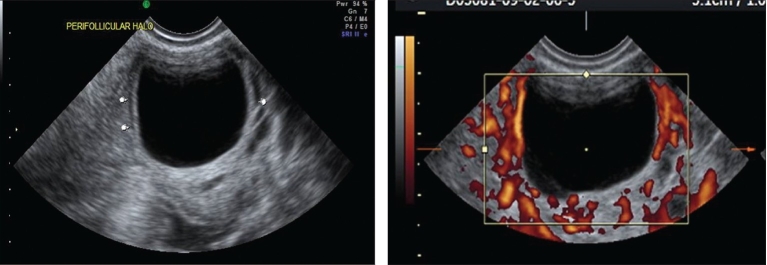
Preovulatory follicle with 2D power Doppler
